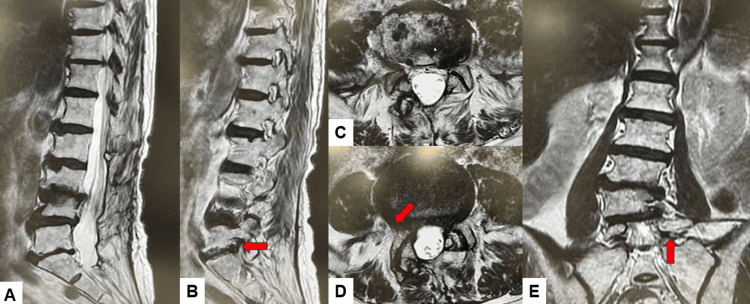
Preoperative MRI. A: Midsagittal T2-weighted image; B: left parasagittal T2-weighted image; C: axial T2-weighted image at the L4/5 level; D: axial T2-weighted image at the L5/S1 level; E: coronal T2-weighted image. Red arrows indicate left L5/S1 foraminal stenosis.
